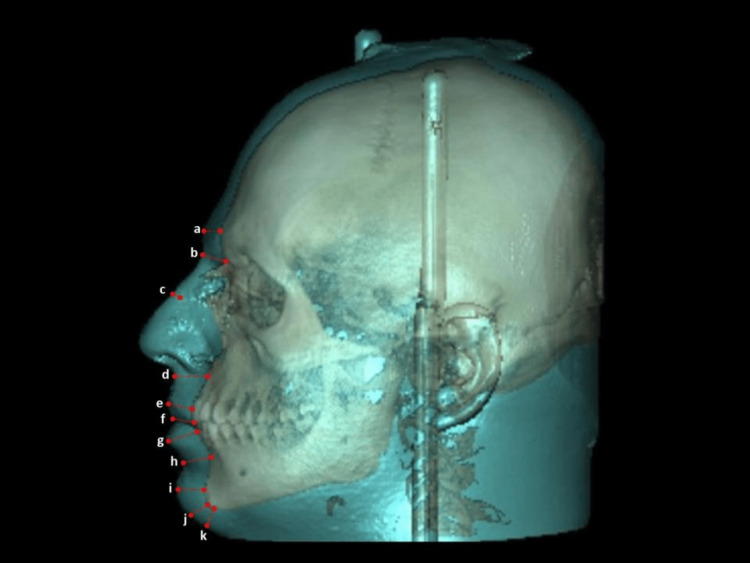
Definitions and measurements of CBCT landmarks in sagittal view (a) hard tissue glabella - soft tissue glabella (GL_h_-GL_s_), (b) hard tissue nasion - soft tissue nasion (N_h_-N_s_), (c) hard tissue rhinion - soft tissue rhinion (Rhi_h_-Rhi_s_), (d) hard tissue subspinale - soft tissue subnasale (Ss_h_-Sn_s_), (e) maxillary incisor - labrale superius (U1-LS_s_), (f) hard tissue incision - soft tissue stomion (Inc_h_-Sto_s_), (g) mandibular incisor - labrale inferius (L1-Li_s_), (h) hard tissue supramentale - soft tissue supramentale (Sm_h_-Sm_s_), (i) hard tissue pogonion - soft tissue pogonion (Pg_h_-Pg_s_), (j) hard tissue gnathion - soft tissue gnathion (Gn_h_-Gn_s_), (k) hard tissue menton - soft tissue menton (Me_h_-Me_s_) CBCT - cone-beam computed tomography
